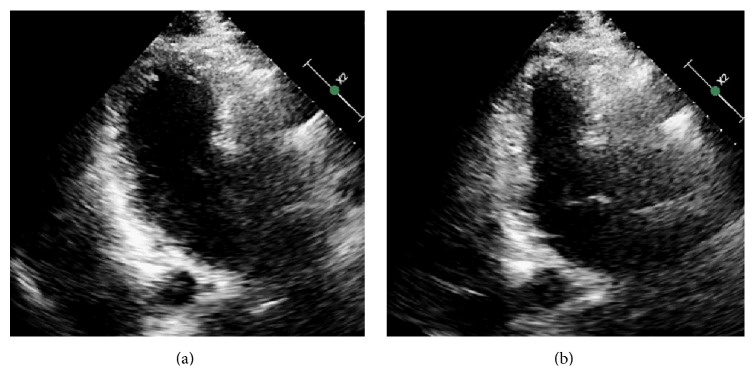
Echocardiographic evidence of normalization of left ventricular ejection fraction. (a) represents diastole and (b) represents systole.
